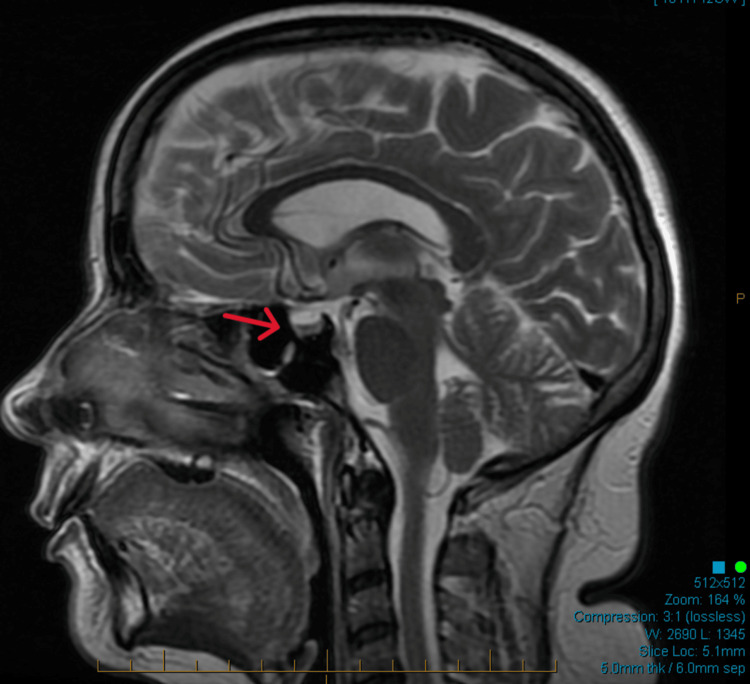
A sagittal brain MRI T2-weighted image showing a normal pituitary gland, which suggests that the gland has the expected size, shape, and signal intensity without abnormalities such as masses or cysts (red arrow) MRI: magnetic resonance imaging
